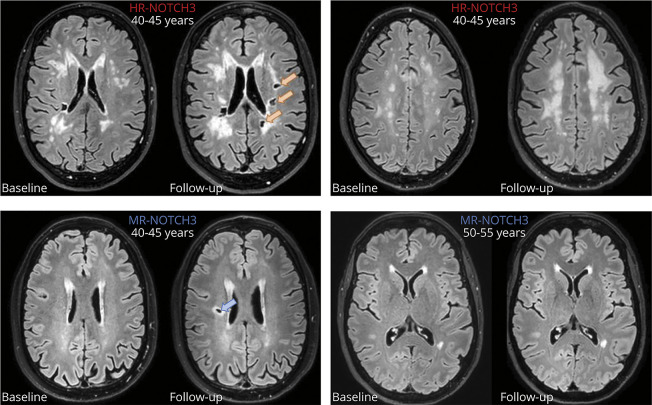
MRI FLAIR Sequences at Baseline and Follow-Up: Examples of Variable Progression of Neuroimaging Markers (A) A patient with an HR-*NOTCH3* variant in their mid 40s developed 12 incident lacunes during the follow-up, of which 3 are shown here (orange arrows); remarkably, this patient did not have any progression of disability on the mRS. (B) A patient with an HR-*NOTCH3* variant in their early 40s whose white matter hyperintensities have increased from patchy to confluent in the semioval center. The patient did not have any incident lacunes, nor did they experience stroke. (C) A patient with an MR-*NOTCH3* variant in their 40s developed 2 lacunes (one of which is indicated with a blue arrow), both in areas with no previous white matter hyperintensities. This patient has a concomitant diagnosis of poorly treated type 1 diabetes. (D) A patient with an MR-*NOTCH3* variant in their early 50s shows a strikingly mild neuroimaging phenotype, with little to no progression with respect to any of the neuroimaging markers. HR-NOTCH3 = high-risk *NOTCH3* variant; MR-NOTCH3 = moderate-risk *NOTCH3* variant, FLAIR: fluid-attenuated inverse recovery; mRS = modified Rankin Scale. To ensure participant anonymity, age ranges are given and sex is not specified.
